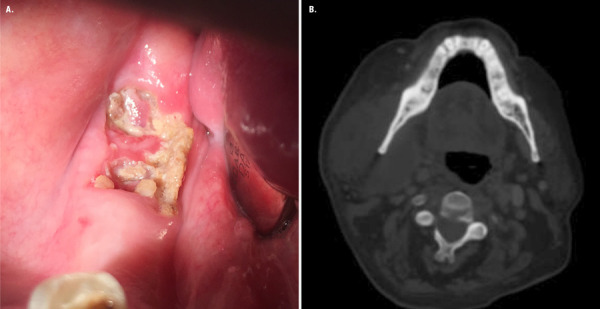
A. Intraoral examination showing necrotic bone exposure in right-side third molar region. B. Axial CT image shows the extension of submandibular space infection on the right-side.
